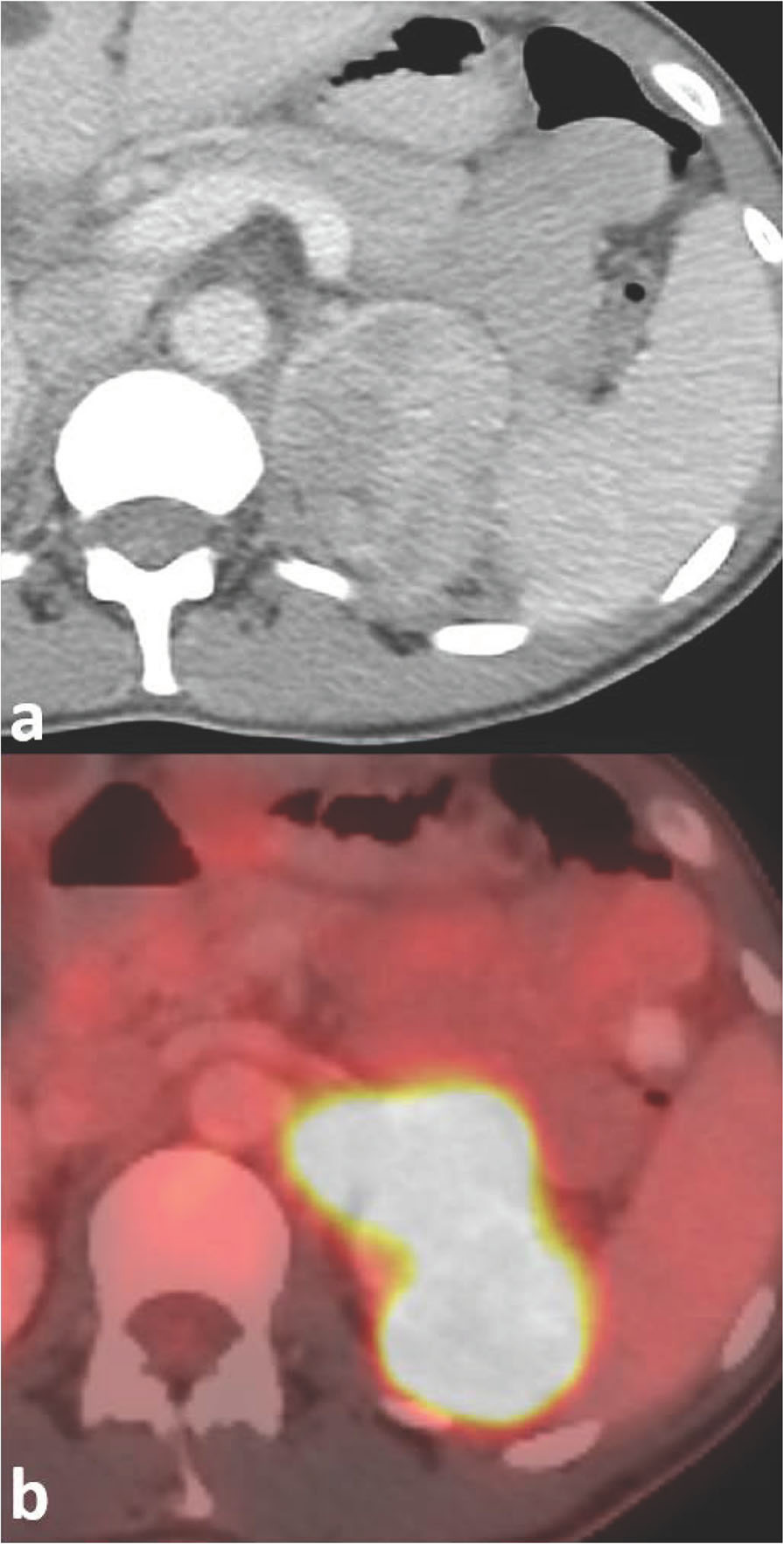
52-year-old male with metastatic fumarate hydratase-deficient renal cell carcinoma. Axial post-contrast computed tomography (a) and axial fused fluorodeoxyglucose (FDG) positron emission tomography/computed tomography (b) show an FDG-avid left renal mass (SUV_max_ 21.9)
